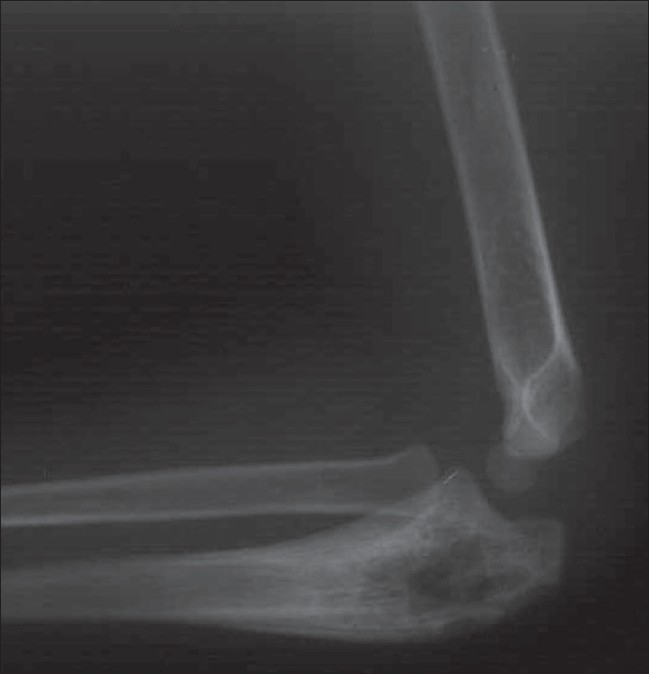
Chronic tuberculous osteomyelitis of the ulna in a child. Plain radiograph of the right forearm shows osteolytic destruction of the proximal ulna with surrounding sclerosis and cortical thickening
